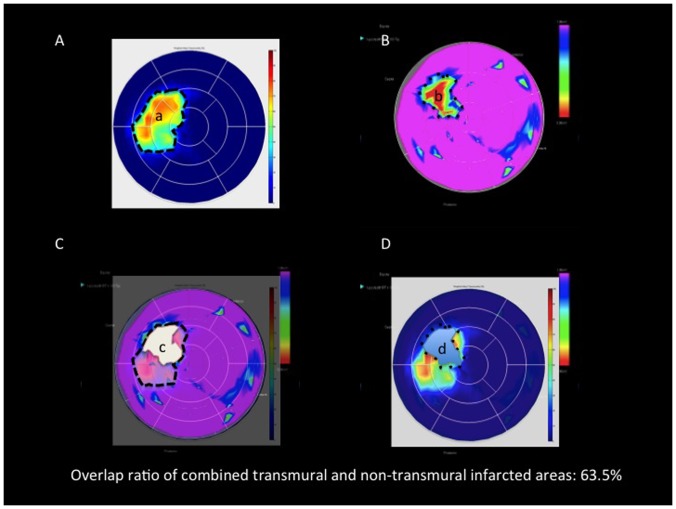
Calculation of the overlapping ratio of the transmural plus the non-transmural infarction by cardiac magnetic resonance imaging (cMRI) and NOGA endocardial mapping. A. cMRI transmurality polar map. B. NOGA bipolar polar map. C and D. Overlap of the cMRI and NOGA polar maps. a: The size of the transmural and non-transmural infarction by cMRI; b: the size of transmural and non-transmural infarction by NOGA bipolar mapping; c: overlap of the NOGA bipolar infarct shape on a cMRI polar map; d: overlap of the cMRI infarct shape on a NOGA bipolar map. The overlapping ratio was calculated as follows: (c+d)/(a+b).
